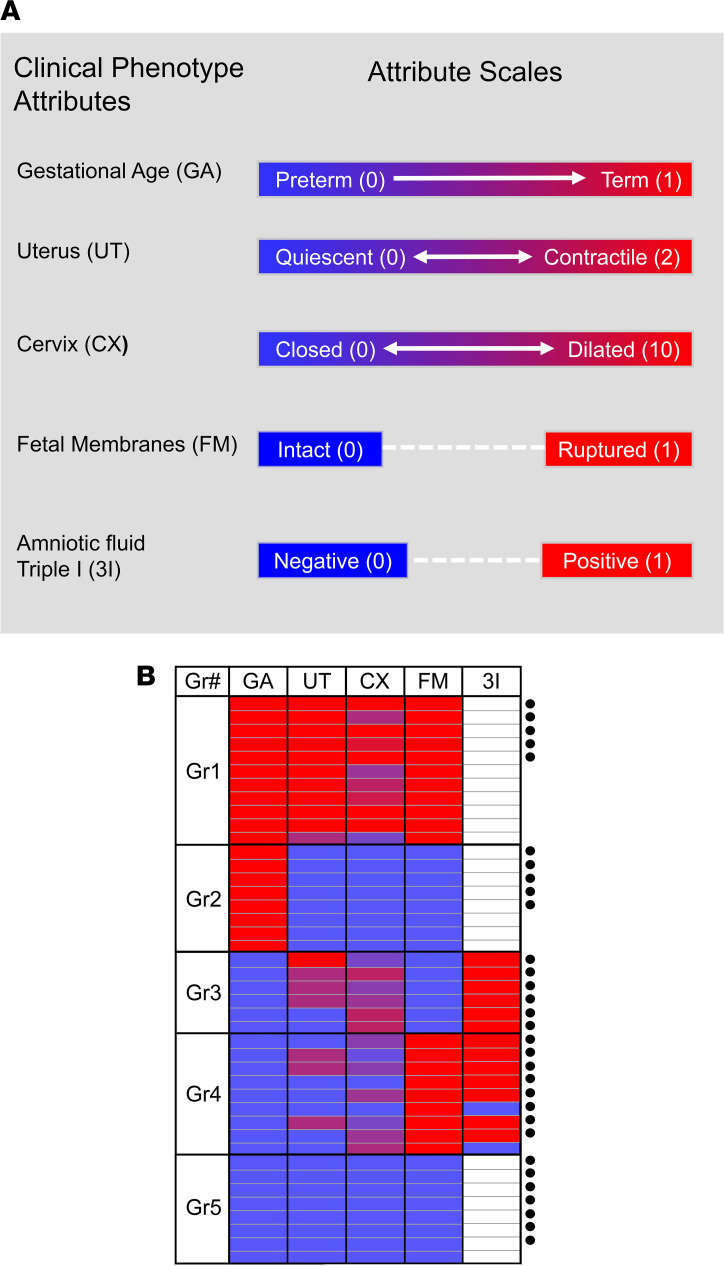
Overview of clinical characteristics of myometrial samples. (A) Graphical summary of clinical phenotypic attributes included as part of the classification algorithm used in this study. Gestational age was established clinically based on last menstrual period and/or ultrasonographic examination prior to 20 weeks (wk) of gestation and dichotomized into term (1, delivery > 37 wk of gestation) and preterm (0, delivery < 34 wk of gestation) cases. Uterine contractions were scored semiquantitatively on a scale from 0 to 2 (0, absent; 1, irregular, not followed by cervical change or when contractions receded after tocolysis; or 2, regular and followed by cervical change). Cervical dilation was scored on a scale from 0 to 10 cm as recorded on the last exam prior to cesarean. Membrane status was scored as 0 (intact) or 1 (ruptured). Triple I was scored as 0 (absent) or 1 (confirmed or suspected). (B) Heatmap of clinical characteristics for all cases included in the current study. Cases were aggregated into 5 groups as follows: Gr1, term birth following spontaneous onset of term labor (TL); Gr2, term birth by elective cesarean section not in labor (TNL); Gr3, PTB following spontaneous preterm labor with intact membranes (PTB-sPTL); Gr4, PTB following PPROM (PTB-PPROM); and Gr5, provider-initiated PTB in the absence of active labor contractions, cervical dilation, or membrane rupture (PTB-PI). Additional phenotyping of cases spontaneously committed to PTBs (Gr3 and Gr4) involved presence or absence of Triple I based on cultures of amniotic fluid obtained via clinically indicated amniocentesis as described in prior studies. The circular symbols to the right of the image denote samples that were included in the exploratory phase of the study.
